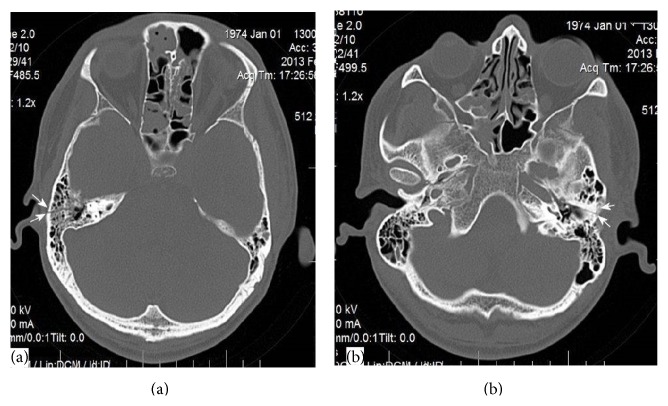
A CT scan performed on admission, showing a right transverse temporal fracture (a) and a left transverse temporal fracture (b).
